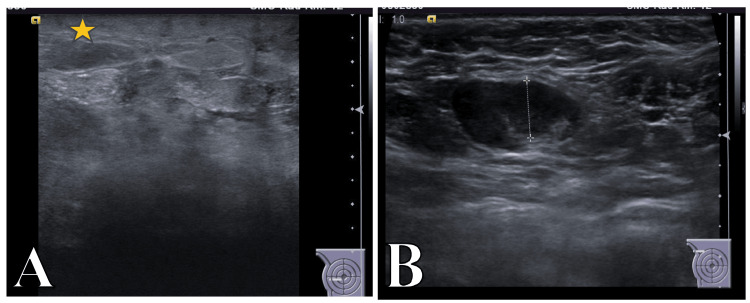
Ultrasound images (A) Ultrasound image of the right breast shows skin thickening (star) with significant subcutaneous edema leading to poor acoustic window and obscuring the underlying breast tissues. (B) Ultrasound image of the right axilla shows an enlarged lymph node with significant cortical thickening.
